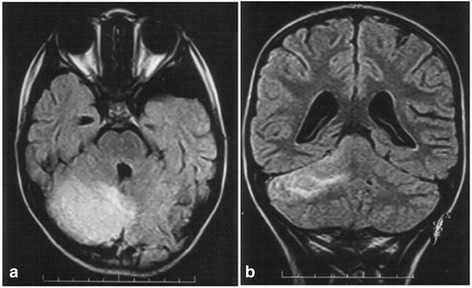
a Flair MRI lesion subcortical white matter right cerebellar hemisphere (case 6). b Flair MRI diffuse cortical lesion right cerebellar hemisphere
